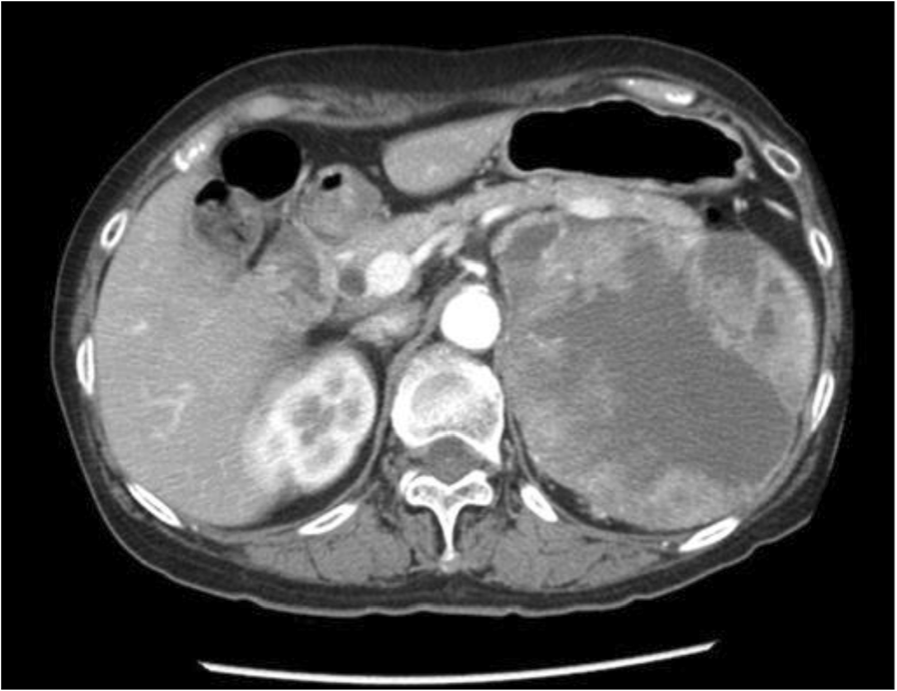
Contrast-enhanced computed tomography examination prior to treatment. A tumor with a 13-cm diameter was detected in the left kidney
